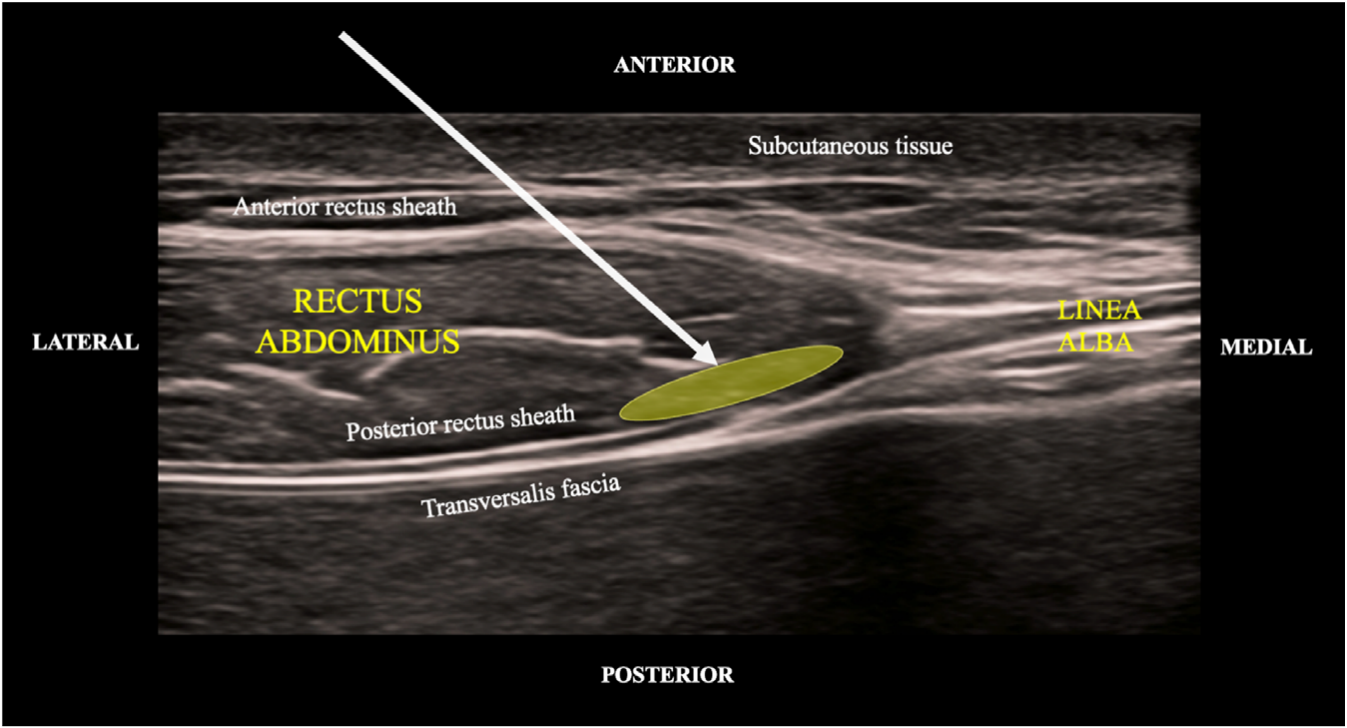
Point-of-care ultrasound demonstrating anatomical landmarks in the rectus sheath block. Yellow oval denotes the target area of injection. Arrow represents relative angle of trajectory for the needle.
